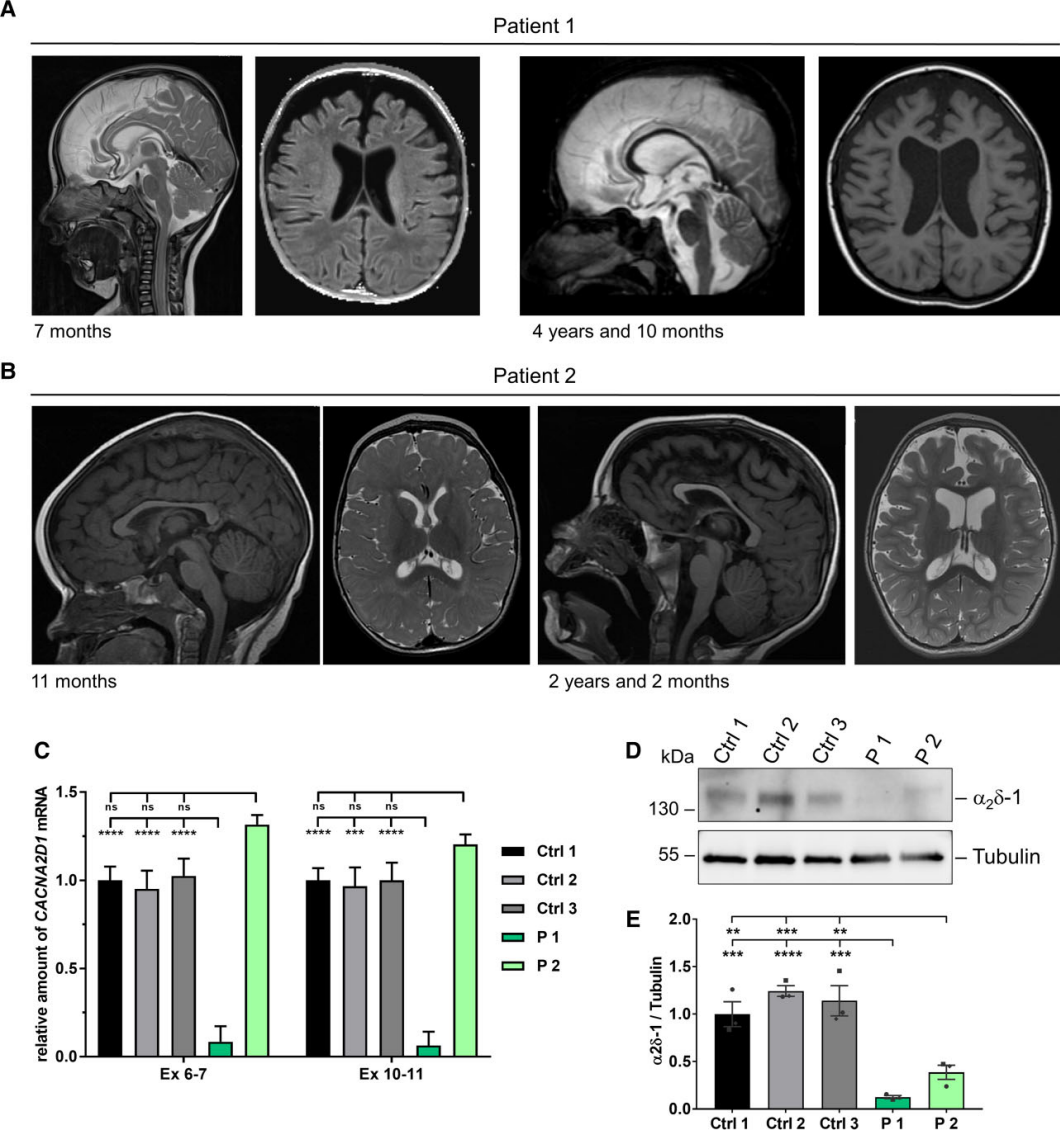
MRI scans of patients with biallelic variants in *CACNA2D1* and determination of *CACNA2D1* mRNA and α_2_δ-1 protein levels in patient-derived fibroblasts. (A) In Patient 1, T_2_-weighted sagittal MRI shows hypoplastic corpus callosum and T_1_-weighted axial image shows enlarged ventricles and frontotemporal CSF spaces at the age of 7 months. At the age of 4 years and 10 months, progressive frontotemporal and mesial temporal atrophy was noted. (B) At the age of 11 months, T_1_-weighted sagittal and T_2_-weighted axial MRI images of Patient 2 show non-specific findings of delayed myelination within the frontal and parietal white matter for age and prominent perivascular spaces. At the age of 26 months, T_1_-weighted sagittal MRI shows interval generalized volume loss with extra-axial spaces and thinning of the corpus callosum. T_2_-weighted axial images show an increase in ventricular size in addition to increased intra-axial spaces. Permission to publish MRI scans was provided for the two patients shown here. (C) Relative quantification of *CACNA2D1* transcripts by reverse transcription-qPCR (RT-qPCR) using two *CACNA2D1*-specific primer pairs generating amplicons for exons 6–7 and 10–11. RNA was obtained from fibroblasts of Patients 1 and 2 and three healthy individuals (Controls 1–3). Glyceraldehyde-3-phosphate dehydrogenase (*GAPDH*) mRNA was used as an internal control; and the amount of target mRNA relative to *GAPDH* mRNA is presented. Mean ±  standard error of the mean (SEM) of three independent experiments, each performed in triplicate, is shown. One-way ANOVA with Bonferroni *post hoc* test for multiple comparisons was used for statistical analysis: ns, *P* > 0.05; P* = 0.0001; *P* < 0.0001. Datasets of independent RT-qPCR experiments with technical triplicates are shown in Supplementary Fig. 2. (D) Representative immunoblot of whole-cell lysates obtained from fibroblasts of Patients 1 and 2 and three controls. The amount of α_2_δ-1 was monitored with an anti-α_2_δ-1 antibody. An anti-tubulin antibody was used to demonstrate equal loading. A band corresponding to α_2_δ-1, which shows a low expression in fibroblasts, was observed in all control cells. Uncropped blots are shown in Supplementary Fig. 3. (E) Band intensities were quantified using the ChemiDoc imaging system. Mean ± SEM with individual data-points of three independent experiments is shown. One-way ANOVA followed by a Bonferroni *post hoc* test for multiple comparisons was used for statistical analysis: *P* < 0.0065; P* < 0.0005; *P* < 0.0001. Ctrl = control; ex = exon; ns = not significant; P = patient.
